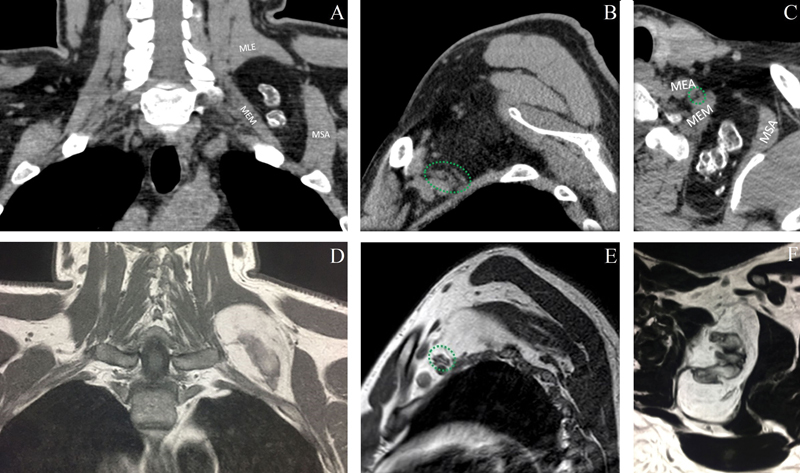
Computed tomography in coronal section in A, sagittal in B, and axial in C, demonstrating expansive formation located in the deep soft parts of the left paravertebral space, with attenuation similar to fat and coarse calcifications inside, in addition to thin septations in contact with the brachial plexus (green circle). Magnetic resonance in coronal cut T1 in D, sagittal T1 in E, and axial T2 in F, demonstrating encapsulated expansive formation located in the deep soft parts of the left paravertebral space with predominance of fat-like signal in all sequences with ossifications inside in contact with the brachial plexus (green circle).
